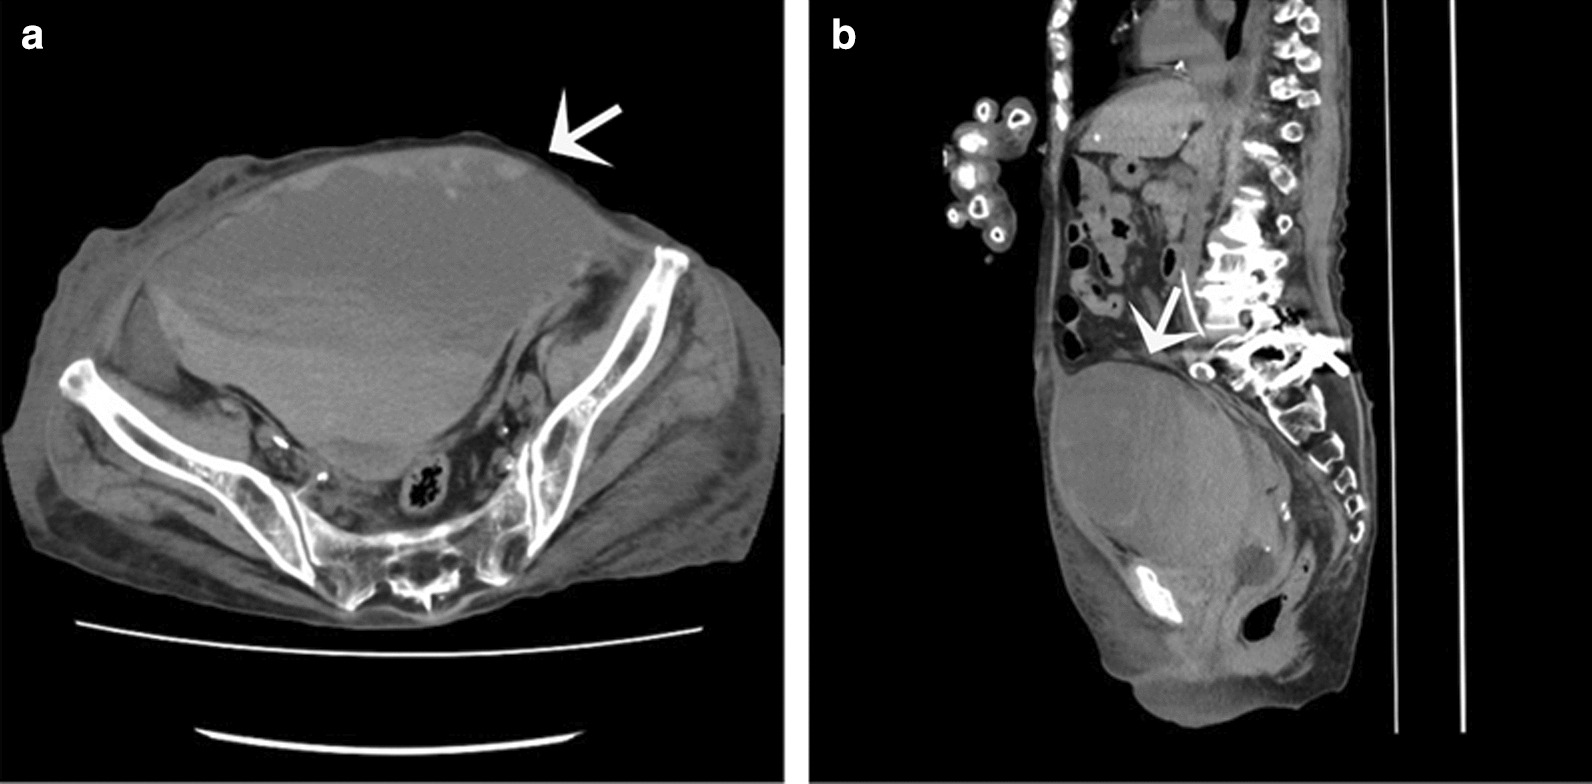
Non-contrast-enhanced abdominal computed tomography (CT) scan at caused abdominal pain. a Axial image. b Sagittal image. CT scan shows a hematoma in the left rectus sheath (arrow)
